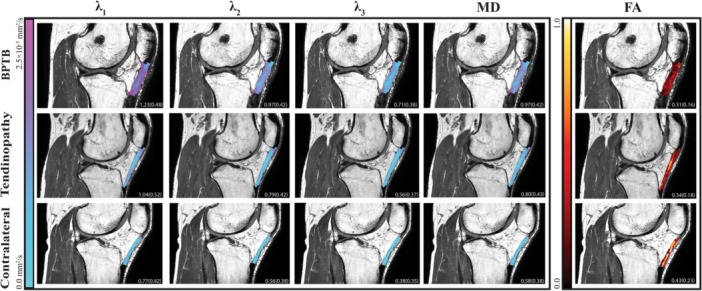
Diffusion scalar maps of diffusivity (λ_1_, λ_2_, λ_3_), mean diffusivity (MD) and fractional anisotropy (FA) measures overlaid with proton density MRI of a patellar tendon 8‐months after bone‐patellar tendon‐bone (BPTB) autograft harvest, a tendon with tendinopathy, and a contralateral patellar tendon. Mean and standard deviation of the scalar metric included in the bottom right corner of each image.
